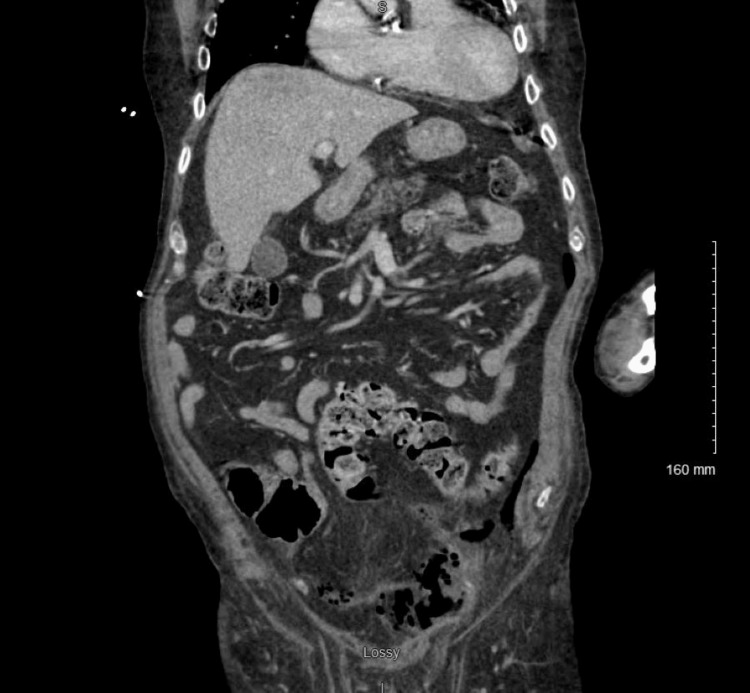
CT abdomen and pelvis with IV contrast demonstrating moderate pneumoperitoneum
